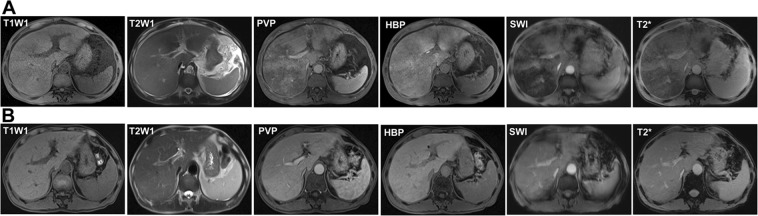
59-year-old man was diagnosed with PAs-induced HSOS after the ingestion of gynura segetum, and he received gadoxetic acid-enhanced MRI scan at the baseline visit and follow-up visit. (A) The images of gadoxetic acid-enhanced MRI, T2*WI and SWI at the baseline visit; (B) the images of gadoxetic acid-enhanced MRI, T2*WI and SWI at the follow-up visit. After effective 3-month treatment, the patients received gadoxetic acid-enhanced MR scan again, and the images showed the abnormality was improved significantly. PVP: portal-venous phase; HBP: hepatobiliary phase.
